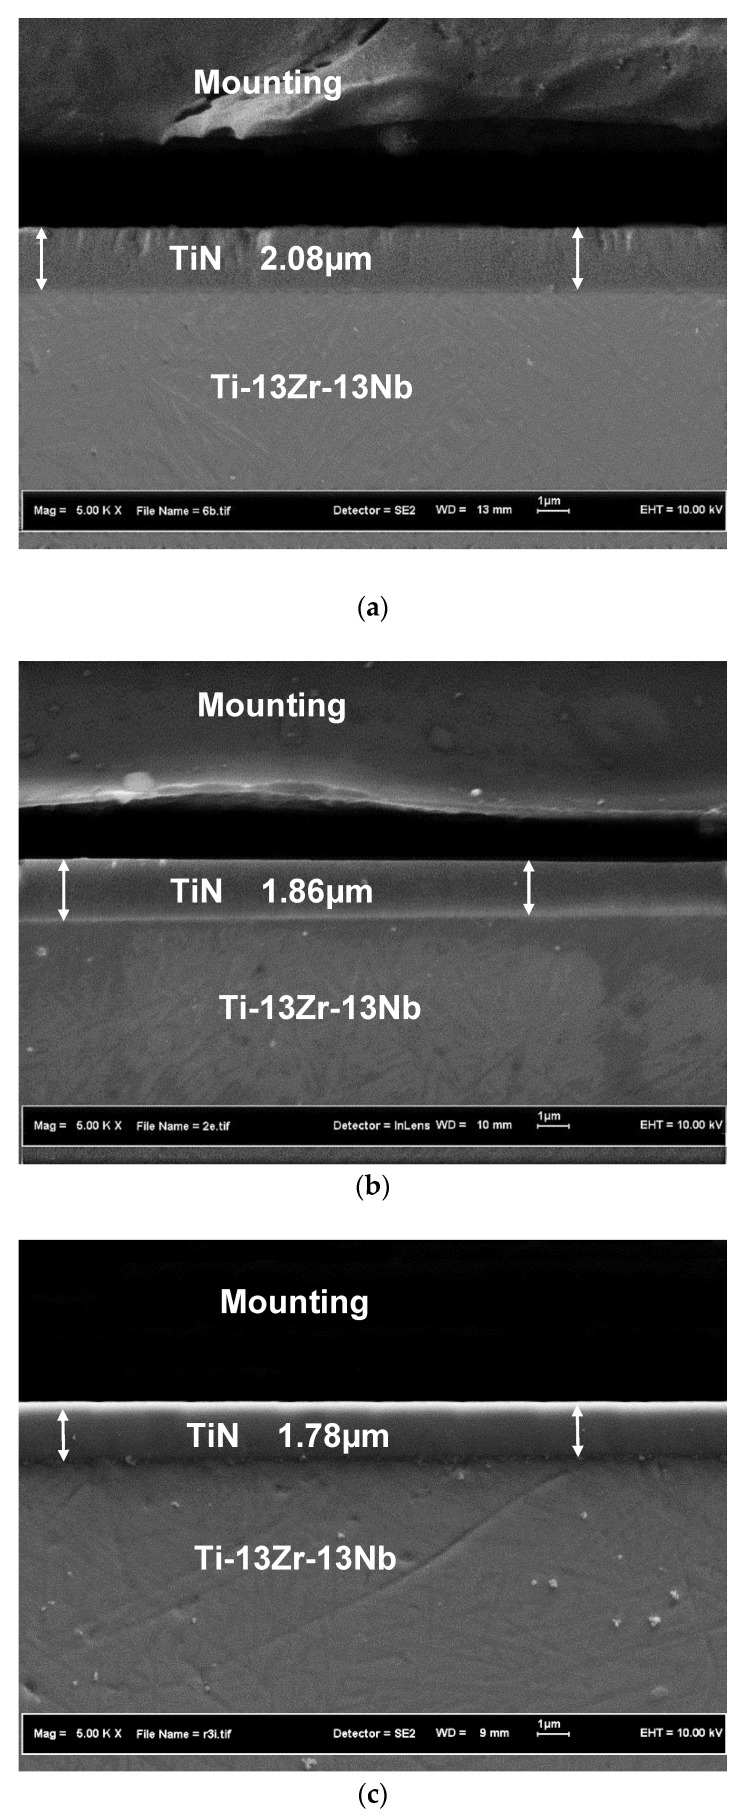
Cross-sectional view of TiN after ultrasonic treatment at 16 kHz for (a) 5 min, (b) 8 min, and (c) 11 min.
